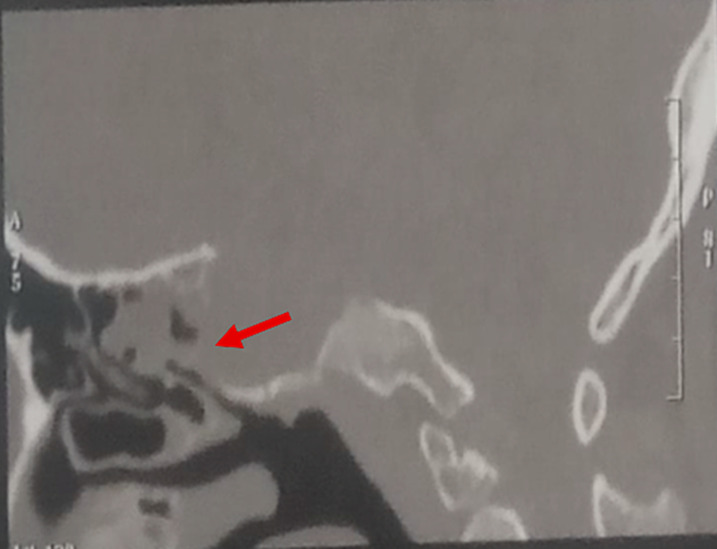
computed tomography scan showing an intra-sellar soft tissue lesion filling the optic chiasm cistern and displacing the optic chiasm superiorly, with involvement of the sphenoid sinus (highlighted by the red arrow)
